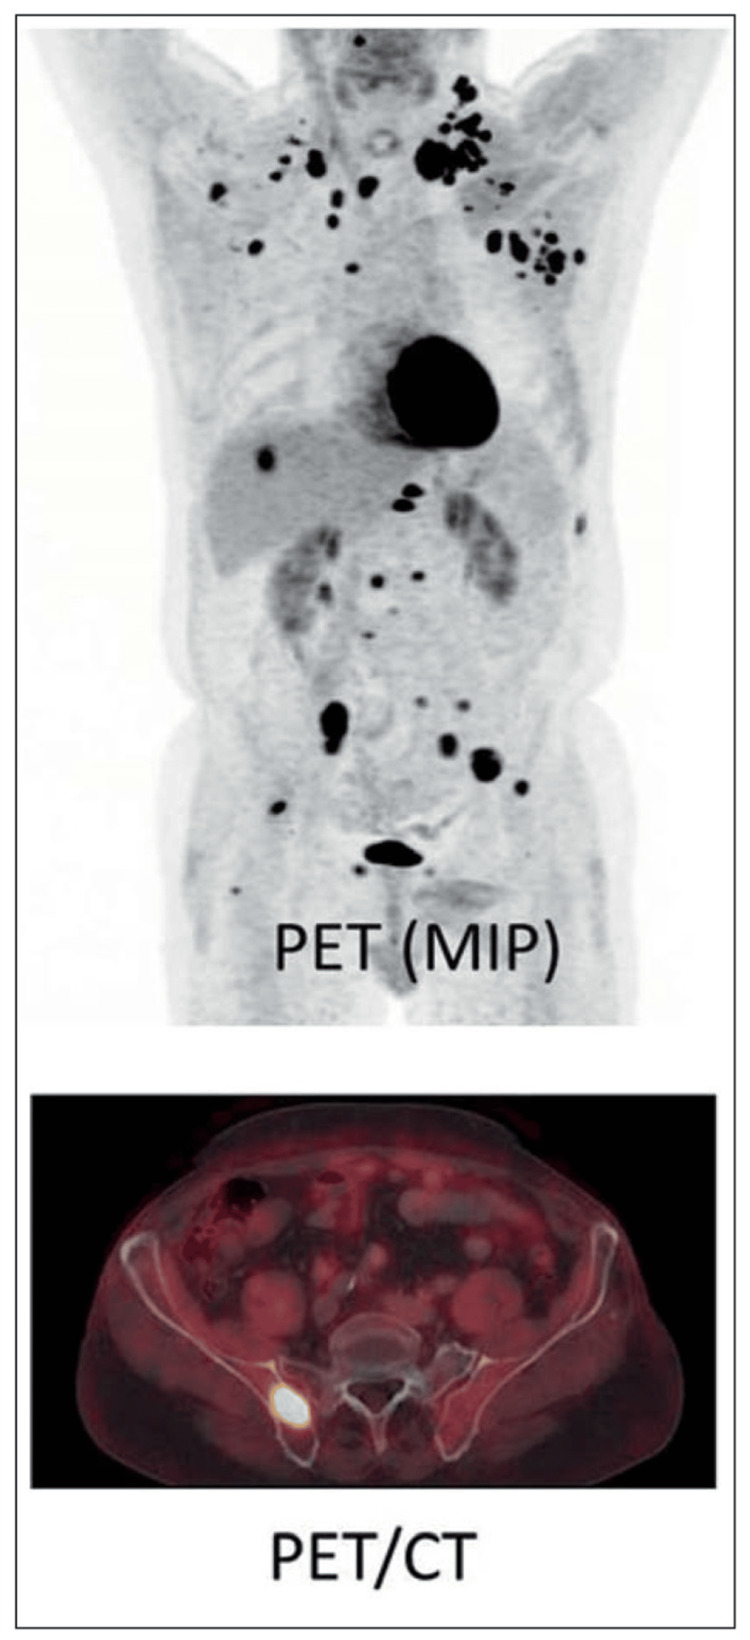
PET and PET/CT imaging demonstrating metabolic tumour burden and systemic disease distribution in skeletal metastasis Source: Heindel et al. [23] Maximum intensity projection (MIP) PET imaging reveals multiple areas of increased radiotracer uptake distributed throughout the axial and appendicular skeleton, indicating widespread metabolically active metastatic disease. The corresponding fused PET/CT image localises areas of high metabolic activity to specific anatomical structures, confirming skeletal involvement and enabling precise lesion localisation. PET/CT demonstrates superior sensitivity in identifying metabolically active lesions that may be inconspicuous on conventional structural imaging. These findings are critical for surgical decision-making, as high systemic tumour burden and multifocal skeletal involvement typically favour palliative stabilisation strategies over aggressive limb-salvage or reconstructive procedures.
